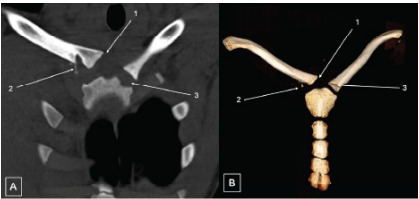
Superior displacement of the right medial clavicle relative to the sternum shown by arrow one on coronal computed tomography (CT) without contrast (A) and three-dimensional CT reconstruction (B). Relative to the displaced clavicle, the epiphyseal ossification is in the expected anatomic location, suggesting fracture of the physis with displacement of the ossified clavicle, shown by arrow two. The unaffected left clavicle is anatomically aligned, shown by arrow three.
